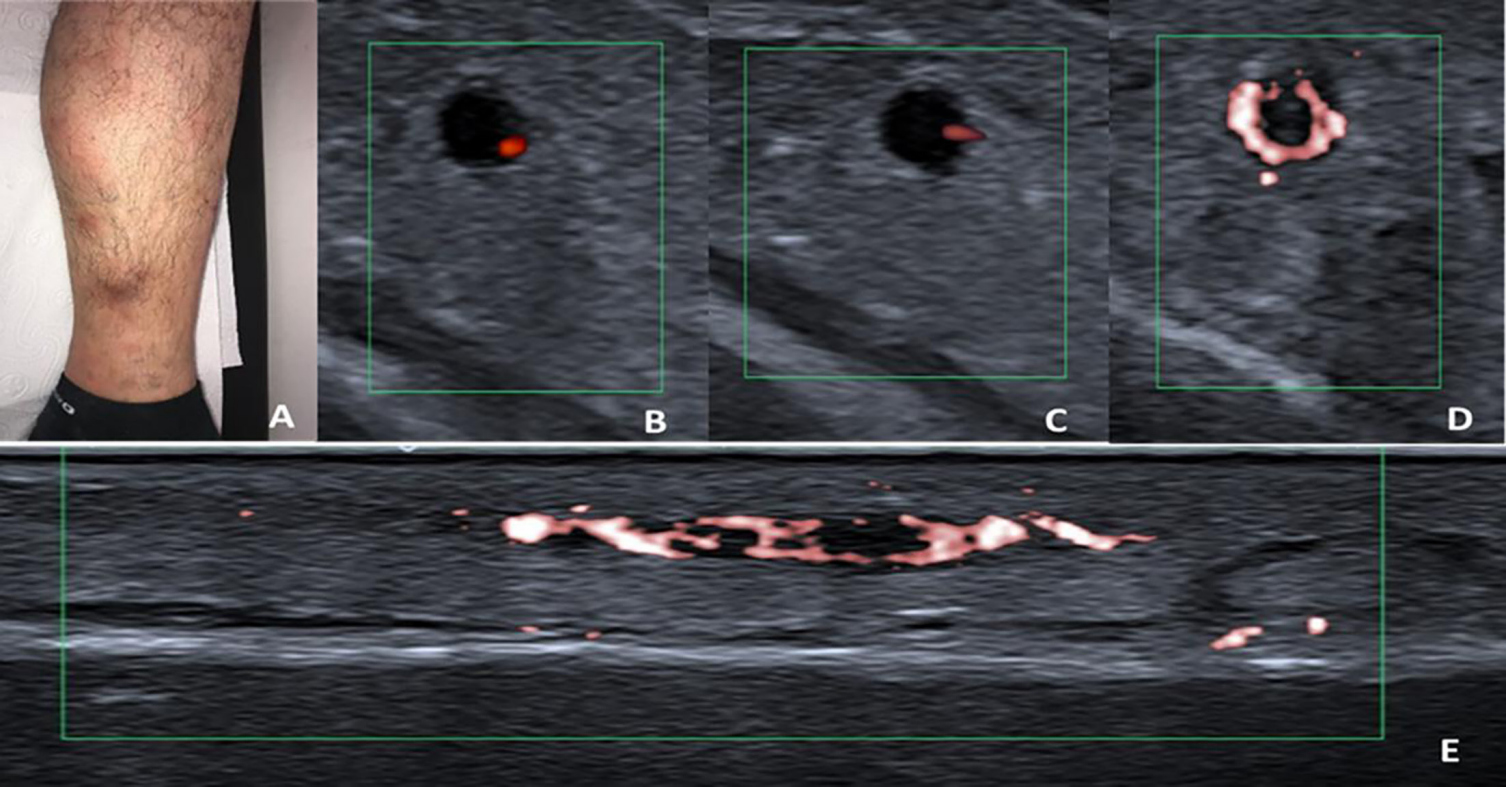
An adult patient with BS presented with acute red, painful nodule-like lesion (A) in the posterior part of the left cruris was evaluated. (B) Colour and (C) power Doppler images show a filling defect compatible with thrombus in the vein, and spot colour coding is seen on the venous vessel wall. (D) On the other hand, SMI image shows grade 4 signal intensity in the axial plane of the venous vessel wall, which suggests vasculitic involvement. (E) In the longitudinal plane, segmental involvement of the venous vessel wall is observed. BS, Behçet syndrome; SMI, superb microvascular imaging.
